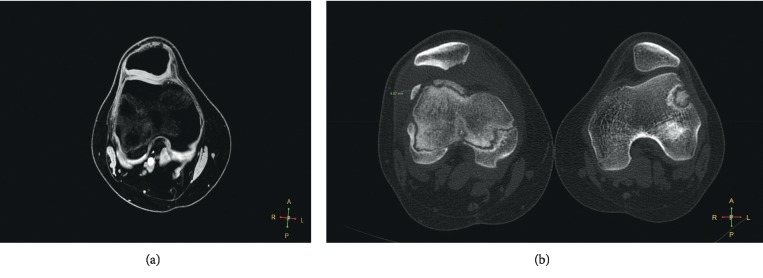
(a) Very good result with perfect OCF healing in follow-up examination in MRI 3D WATS. (b) CT scan preoperatively.
